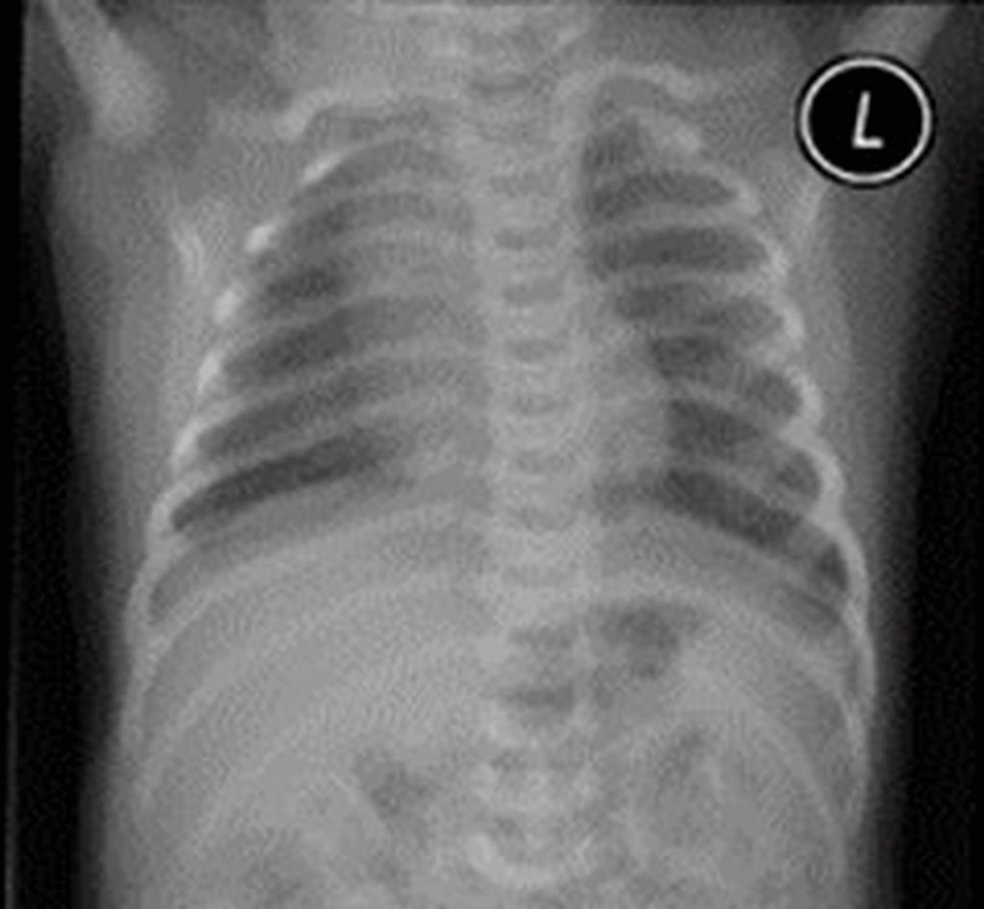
Normal chest X-ray with normal cardiac shadow
